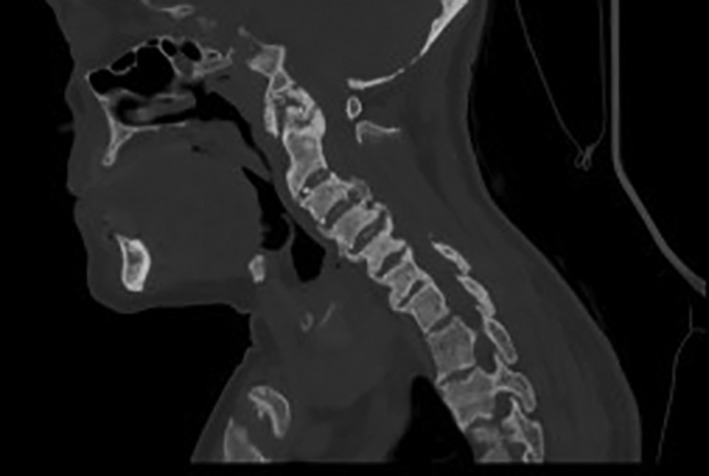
Postoperative CT scan of the cervical spine (March 2019). Sagittal sections: sequelae of posterior laminectomy, decrease in density and thickness of anterior and posterior discal‐ligamentous ossifications.
